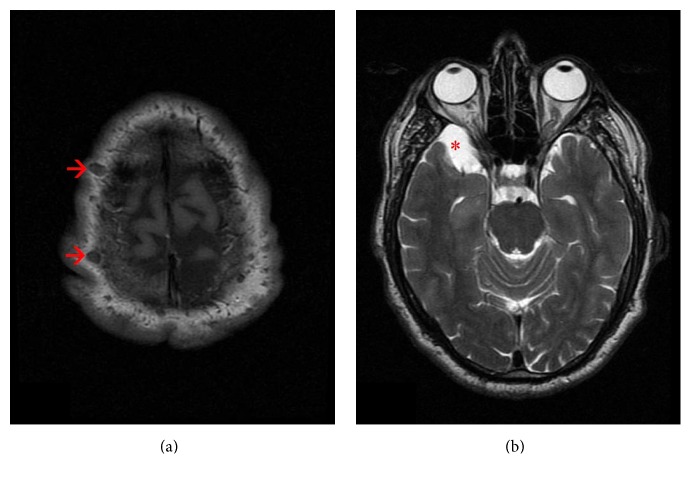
Cranial MRI findings. (a) Axial T1-weighted image depicts multiple neurofibromas on the scalp (marked by arrows). (b) Axial T2-weighted image demonstrates an arachnoid cyst in the right anterior temporal lobe (marked by an asterisk).
